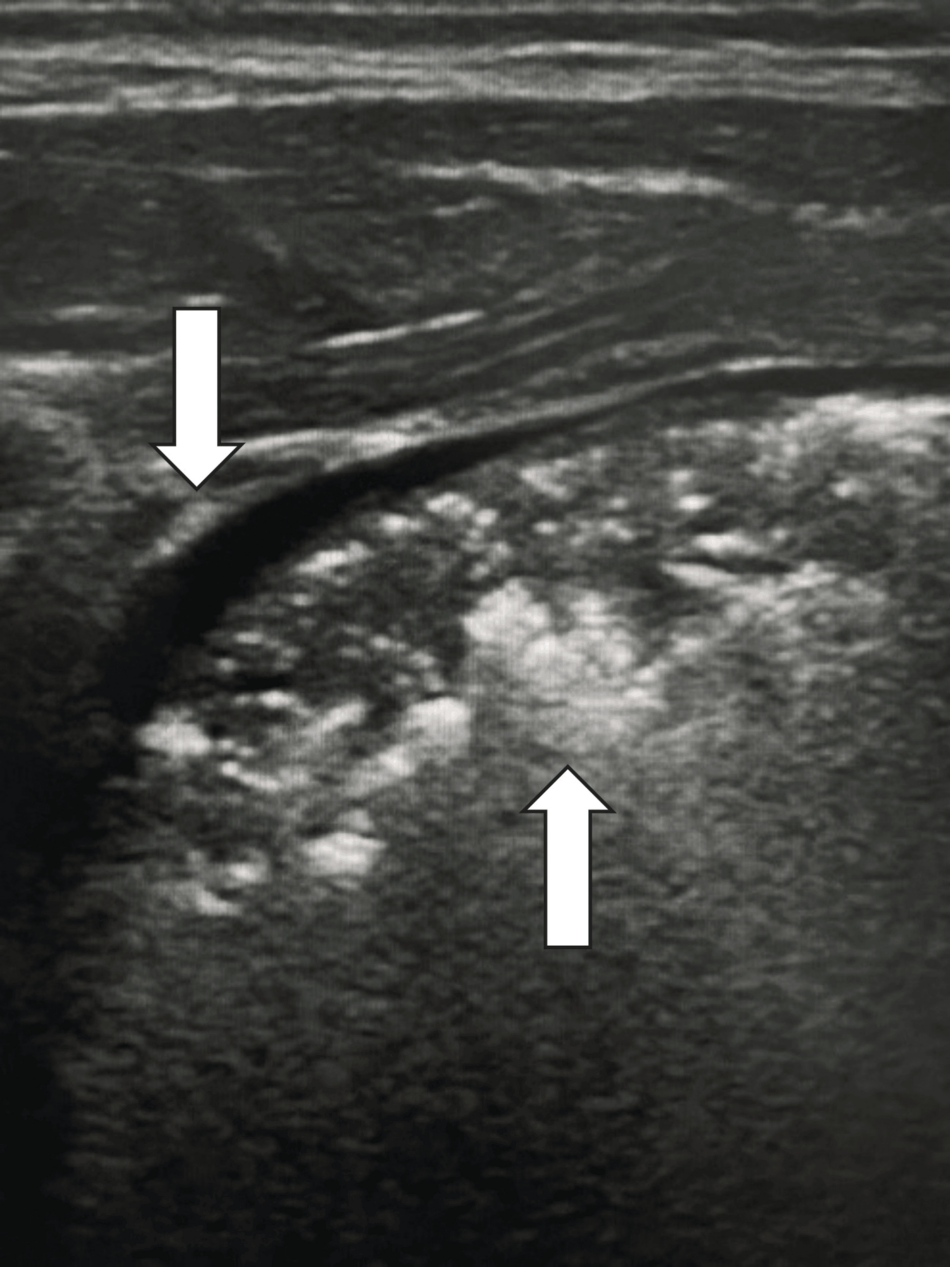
Neonate with meconium aspiration syndrome The lung ultrasound demonstrates a consolidated region in the posterior part of the right lung of a newborn baby, indicated by the lower arrow, 60 minutes after delivery. The ultrasonographic findings consist of loss of the A-line pattern, air bronchograms in the consolidated area, and a pleural effusion between the pleural and the consolidated area, indicated by the upper arrow.
